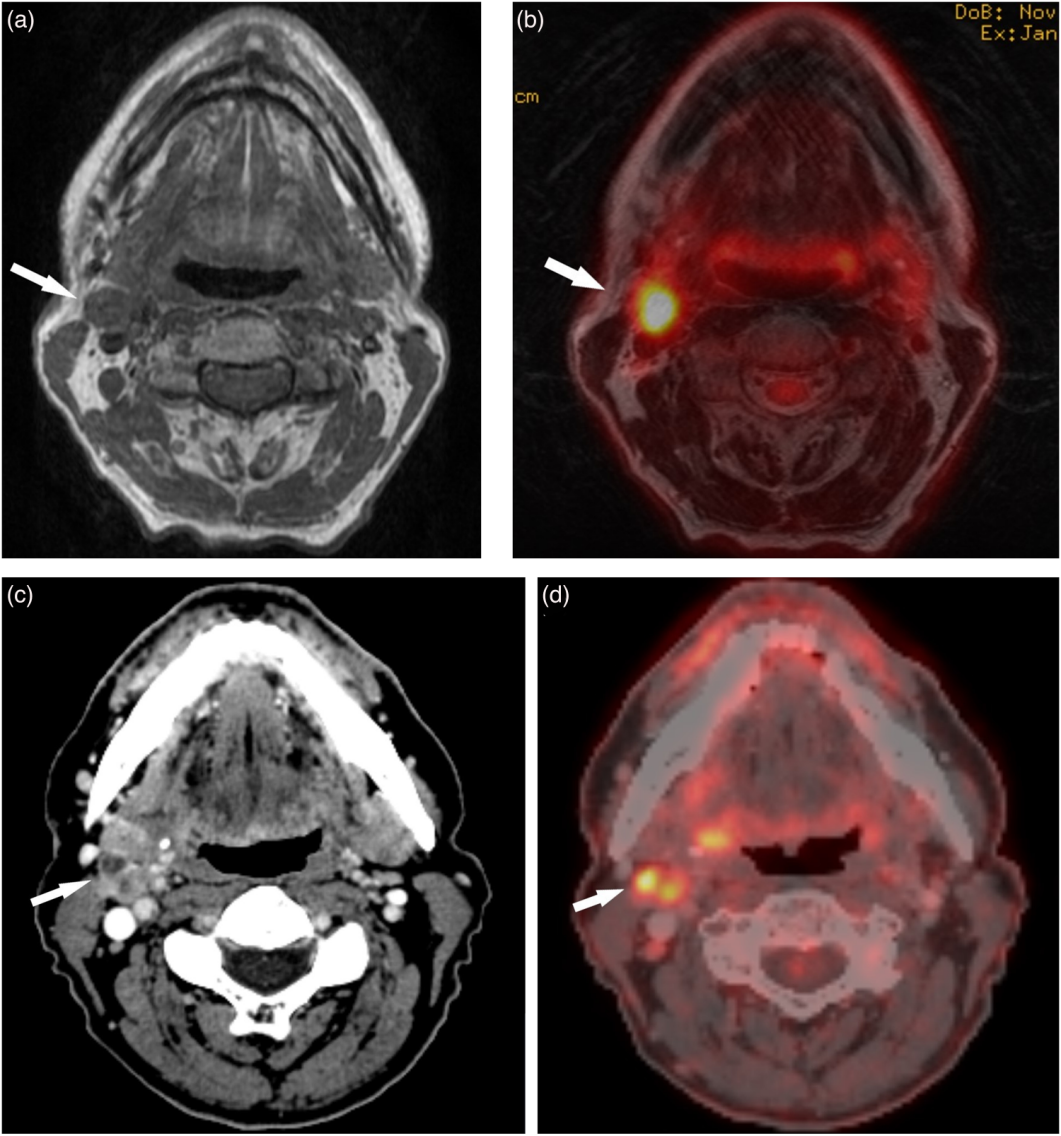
A patient with SCC in the right tonsil and unilateral metastatic nodal conglomerate. (a, b) T1W and T2W PET/MRI hybrid image demonstrate a metastatic node (arrows) with elevated FDG uptake (SUV_max_ 9.6) in the right jugulodigastric area. (c, d) CT and PET/CT hybrid image reveals a conglomerate of metastatic nodes (arrows) with elevated FDG uptake (SUV_max_ 9.6) in the same position. The PET/CT findings were later confirmed by US-FNAC. CT, computed tomography; MRI, magnetic resonance imaging; PET, positron emission tomography; SCC, squamous cell carcinoma; T1W, T1-weighted; T2W, T2-weighted; US-FNAC, ultrasound-guided fine-needle aspiration cytology.
